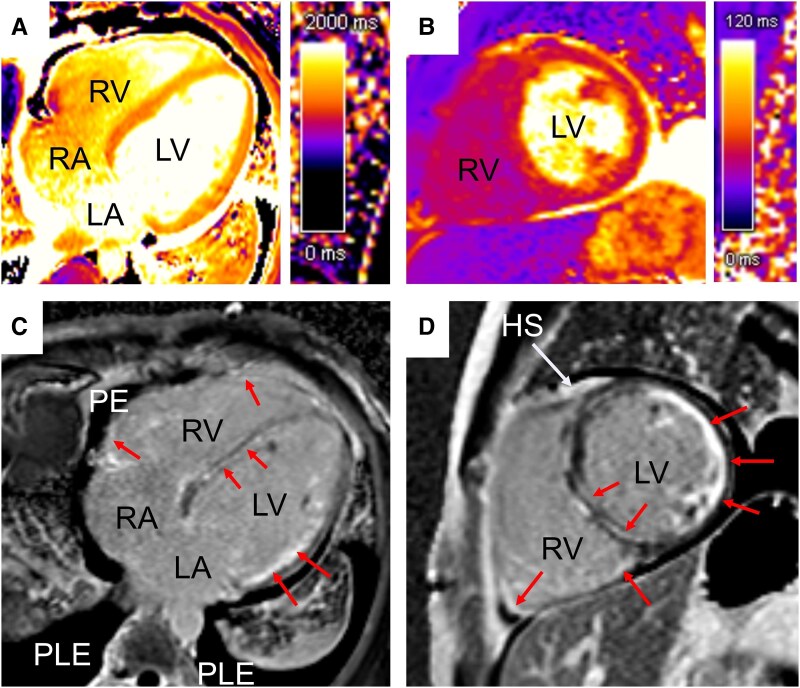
Initial cardiac magnetic resonance imaging. Cardiac magnetic resonance imaging showed ubiquitously increased T1 (*A*: T1 native: 1489 ms; ECV: 41.2%) and T2 values (*B*: 50 ms). Furthermore, multifocal late gadolinium enhancement (*C*, *D*: arrows) including the septal and lateral region as well as the anterior insertion point, extending into the right ventricular wall (*D*: ‘hook sign’), was seen in phase-sensitive inversion recovery imaging. HS, hook sign; LA, left atrium; LV, left ventricle; PE, pericardial effusion; PLE, pleural effusion; RA, right atrium; RV, right ventricle.
